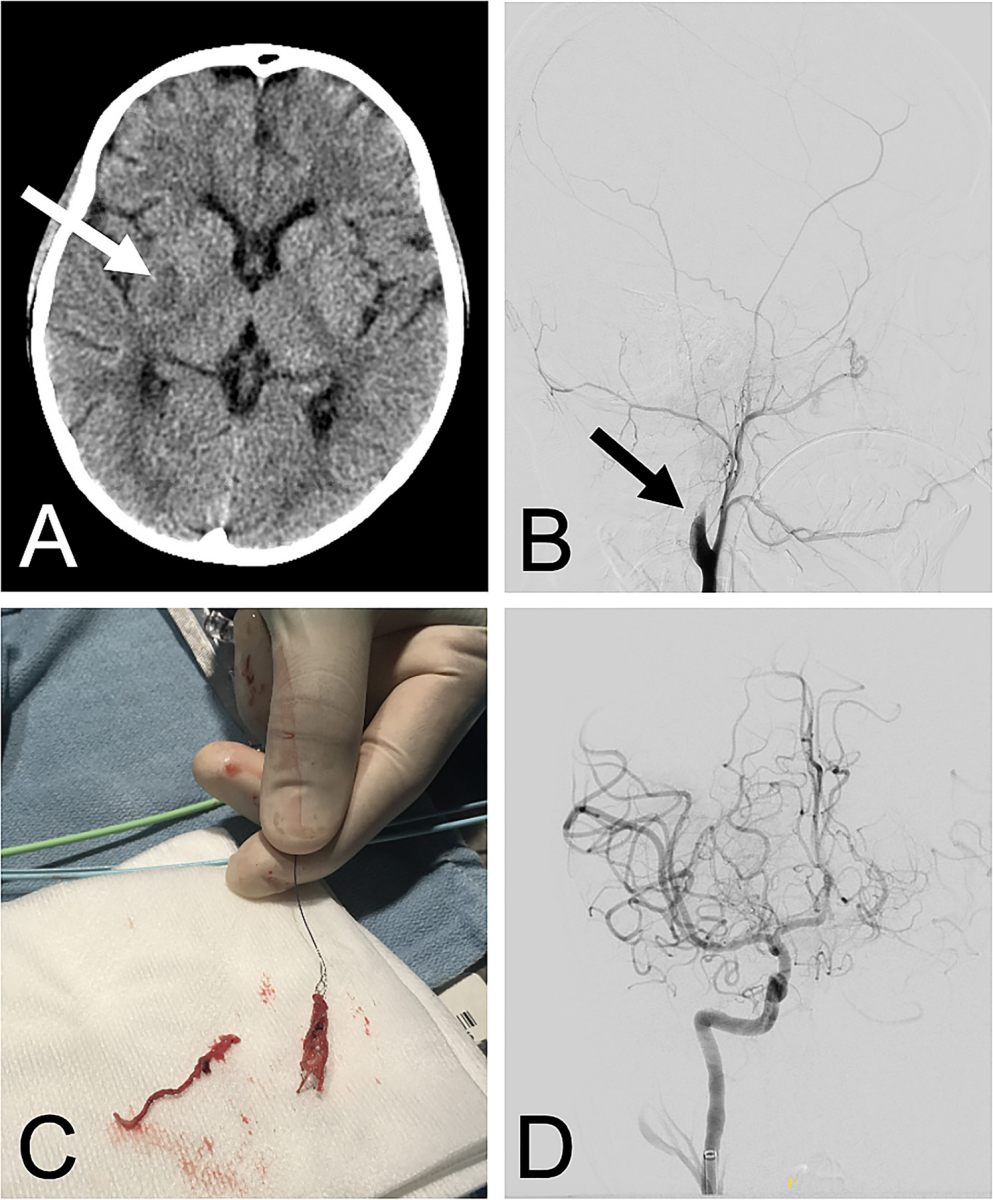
(A) Computed tomography with angiography showing an established right basal ganglia infarct; (B) initial digital subtraction angiography demonstrating absence of flow distal to the right carotid bifurcation; (C) stent retriever with a fresh clot embedded and a second tubular thrombus laying on the side; (D) post thrombectomy digital subtraction angiography confirming complete recanalization of the occluded circulation (color images available in online version).
